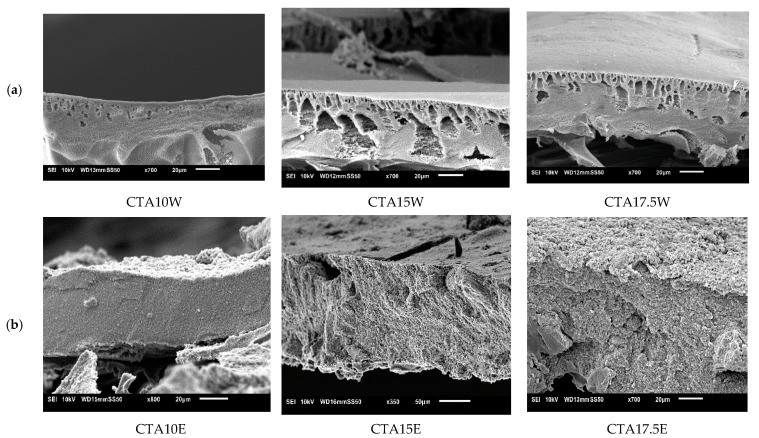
Influence of CTA concentration on SEM cross-sections of CTA membranes cast from using (a) water as non-solvent and (b) ethanol as non-solvent.
